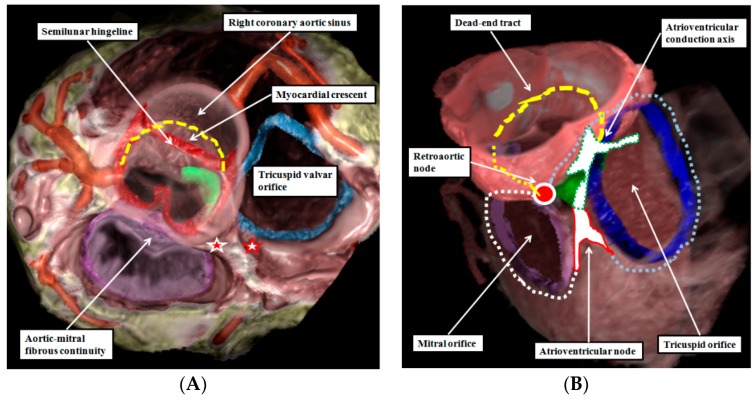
The images are taken from a dataset prepared from a patient undergoing investigation for coronary arterial disease using multi-detector computed tomography. The dataset has been reconstructed to show the semilunar hingelines of the aortic valve in red, the annulus of the tricuspid valve in blue, and the location of the membranous septum in green. The reconstruction is viewed from the cranial aspect in the left hand panel (A), with the left side of the heart seen to the left hand of the observer. The location of the dead-end tract is marked by the yellow dashed line. The white star with red borders shows the location of the atrioventricular node, while the red star with white borders shows the location of the retroaortic node; The right hand panel (B) shows the same reconstruction viewed obliquely from above and from the right. In this view, the location of the atrioventricular node is shown by the while area with red borders, with the atrioventricular conduction axis shown as the white area with dotted green borders. The tricuspid ring is shown by the blue dotted line, and the mitral ring by the yellow dotted line. The dead-end tract is again shown by the yellow dashed line, with the “missing” part of the initial interventricular ring in the area of aortic-to-mitral fibrous continuity shown by the dotted yellow line. The view of the reconstruction in the right hand panel shows how the atrioventricular node and the retroaortic node, shown by the red circle with white borders, are formed at the intersections of the atrioventricular and interventricular rings.
